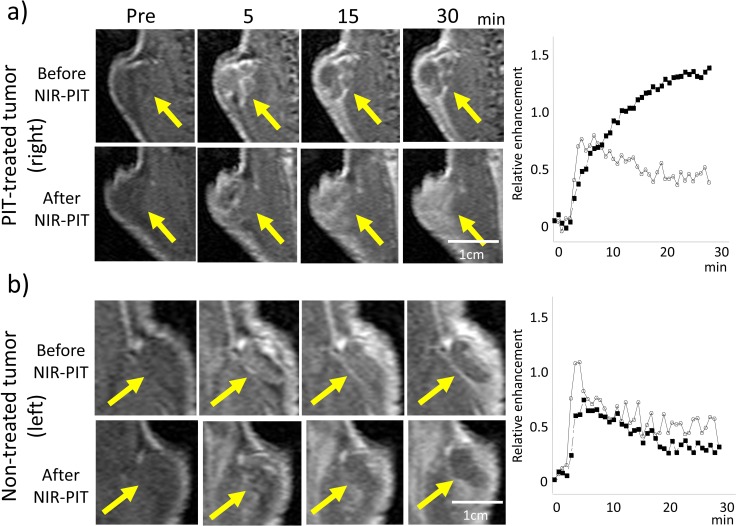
Dynamic MR images after injection of gadofosveset in a NIR-PIT-treated a. or non-treated control tumor b. before and after NIR-PIT (pre-injection, and 5, 15, and 30 min after gadofosveset injection). Arrow indicates the tumor. Before NIR-PIT the treated tumor enhanced immediately after gadofosveset injection and contrast enhancement decreased gradually. After NIR-PIT contrast enhancement of the treated tumor increased gradually until 30 min after gadofosveset injection. On the other hand, contrast enhancement of the non-treated tumor after NIR-PIT seemed to decrease slightly compared to the tumor before NIR-PIT. Time enhancement curve of the NIR-PIT-treated tumor (a: right panel) and the non-treated tumor (b: right panel) using gadofosveset (before (open circles) and after NIR-PIT (filled squares)).
